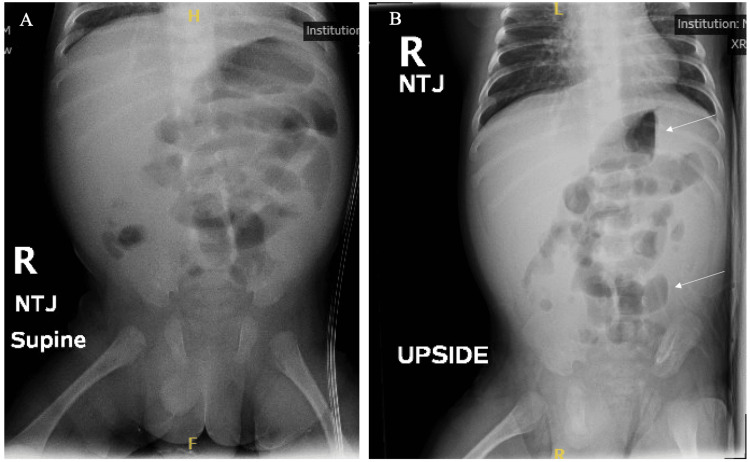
Abdominal X-rays. Initial supine (A) and lateral decubitus (B) abdominal x-rays demonstrated mildly distended proximal bowel and air fluid levels in a pattern suggesting ileus, enteritis, or possible developing obstruction.
